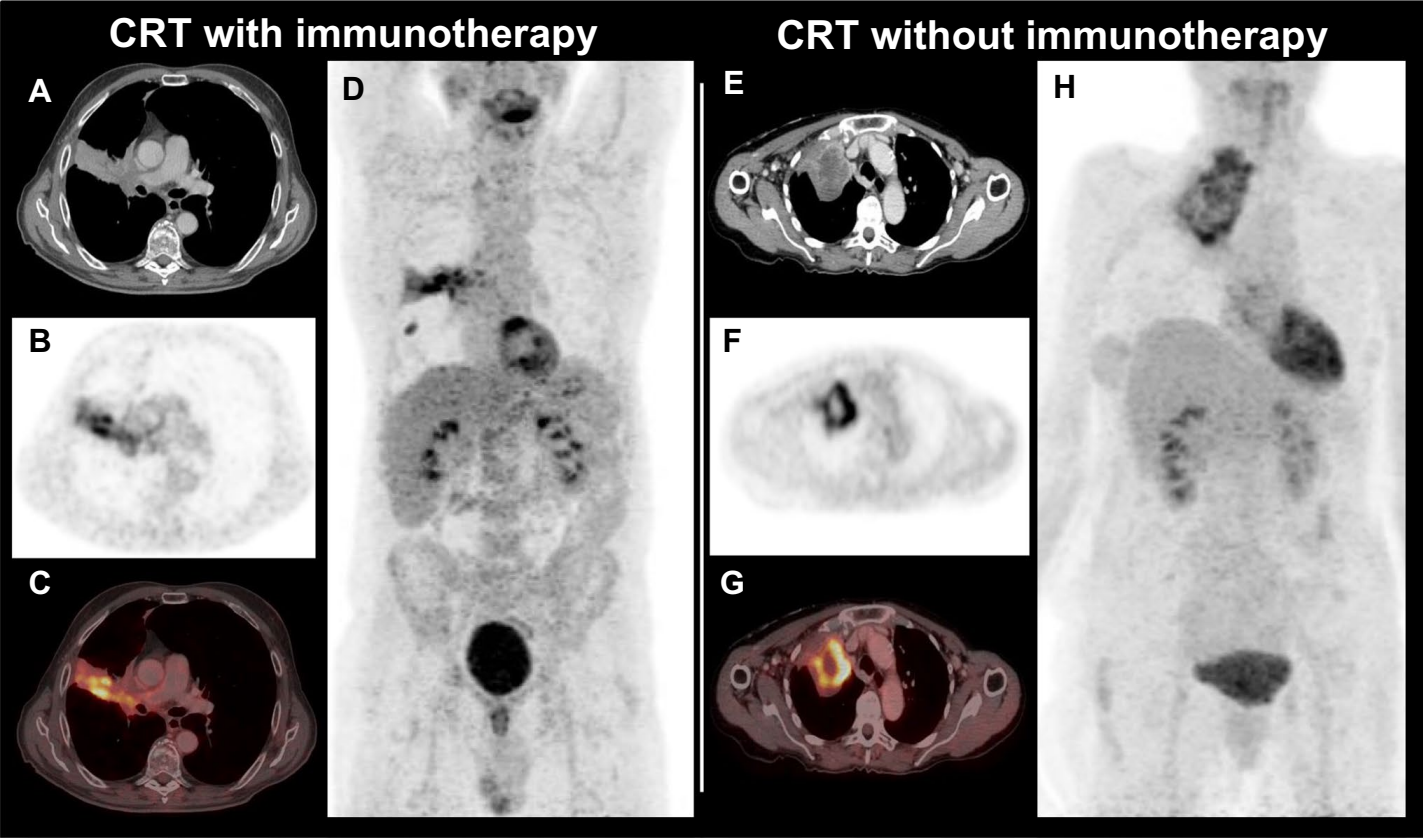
Left: NSCLC patient with CRT-IO and rMTV of 124.0 ml, but OS of at least 43.6 months (still during follow-up). Right: NSCLC patients with CRT only and rMTV of 116.0 ml, but comparably low OS of 8.0 months. A/E, contrast-enhanced CT; B/F, ^18^F-FDG PET; C/G, fused PET/CT; D/H, maximum intensity projections (MIP)
